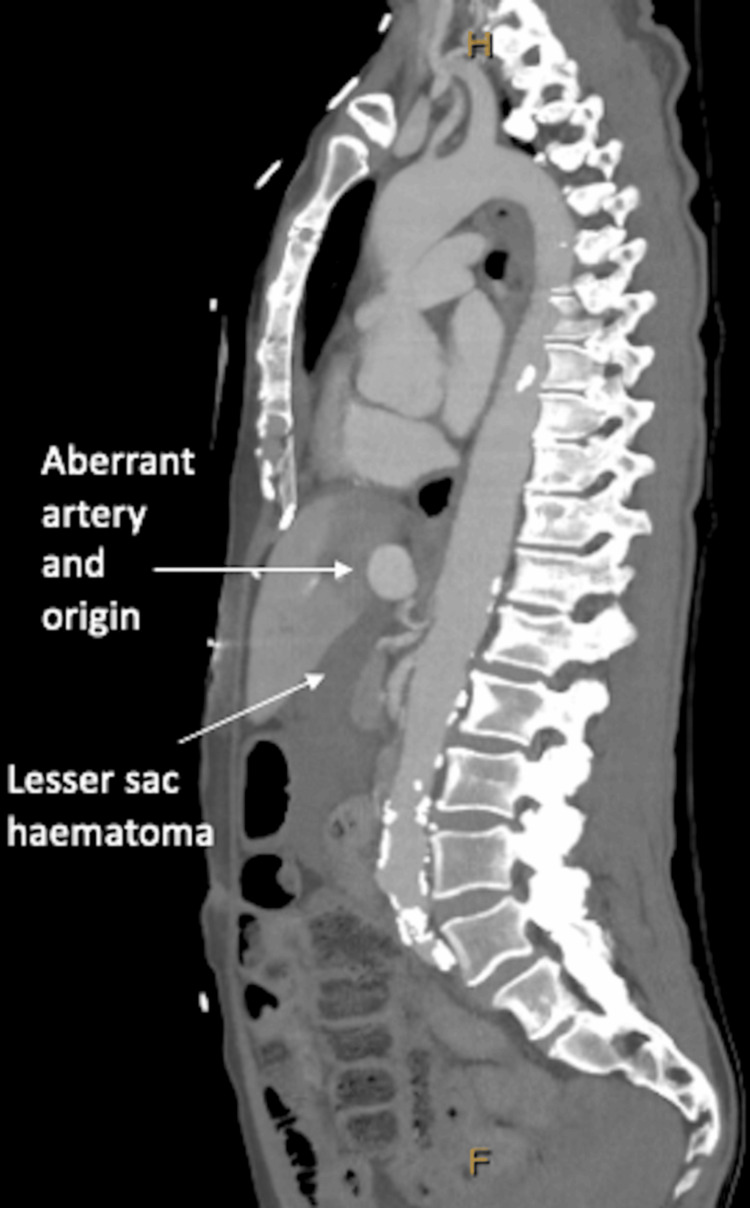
Sagittal phase of CT aortogram demonstrating aberrant left hepatic artery aneurysm and origin from left gastric artery, adjacent to coeliac axis. There is a large haematoma in the lesser sac.
